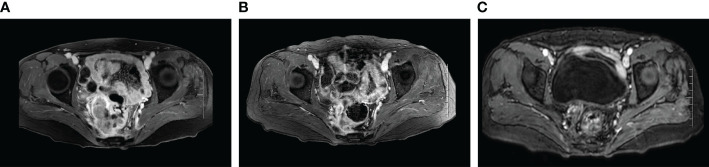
MRI images of local recurrent lesions during treatment. (A) Local recurrence of the last progression (January 2019). (B) MRI images after the last 6 cycles of non-platinum-based chemotherapy combined with bevacizumab (July 2021). (C) MRI images of 12 months after maintenance treatment using the PARP inhibitor Olaparib (July 2022).
